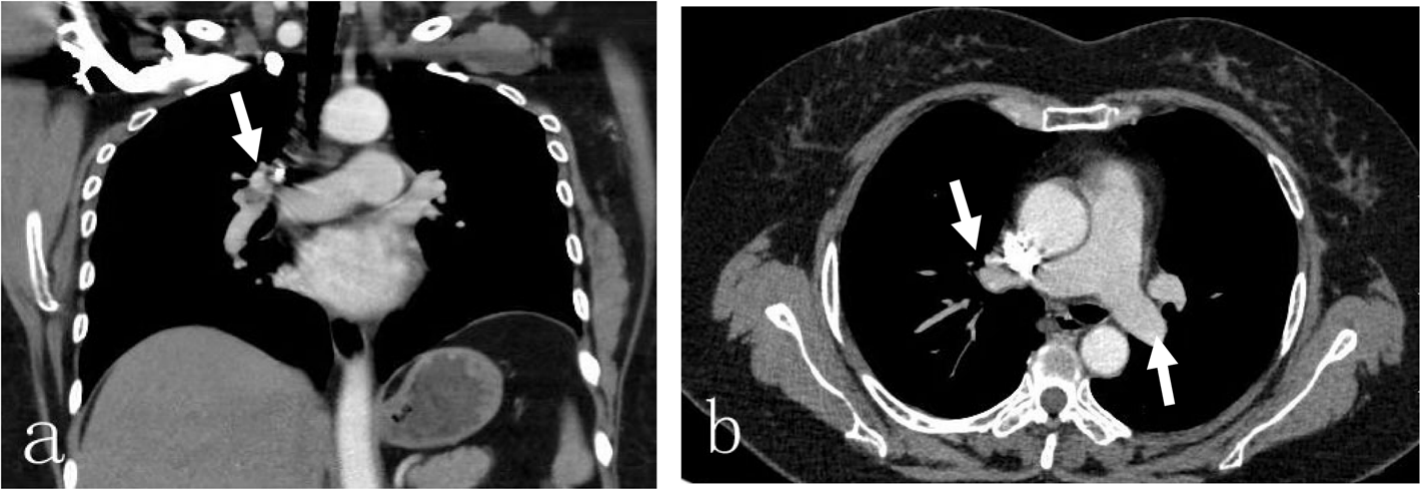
Pulmonary CTA was performed again on the 6th day after thrombolysis therapy and indicated that the pulmonary thrombi in both lungs were significantly reduced compared to those in the previous examination, with partial revascularization and clear pulmonary vascular lines, a coronal view showing a small number of PEs still in the right main pulmonary artery (white arrow), b axial view showing no new PEs in the left and right main pulmonary arteries (white arrow)
